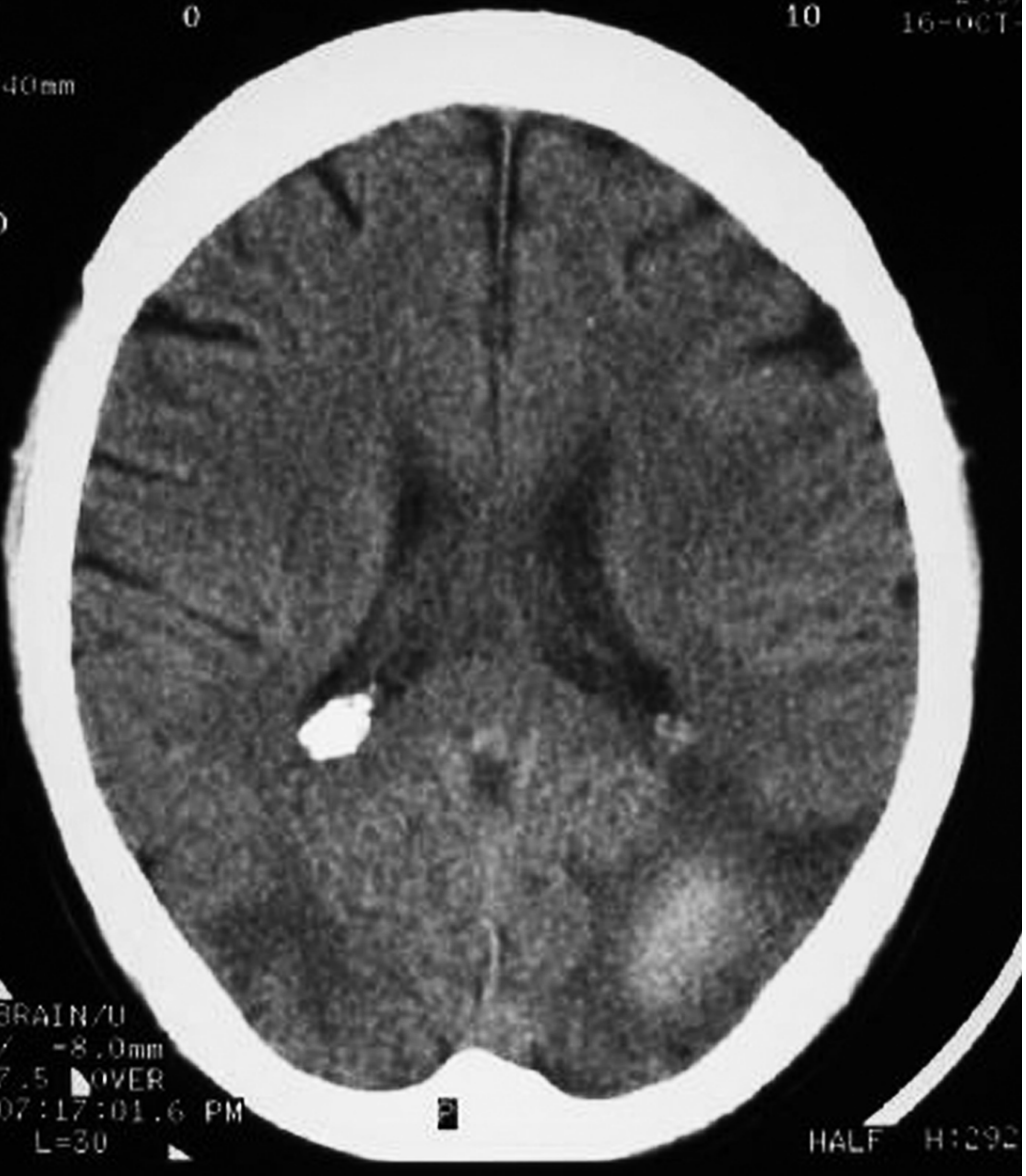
Brain CT without contrast. Decreasing hypodensity in left occipital and parietal lobes with improvement of hemorrhagic foci and almost complete resolution of right occipital lesions.
